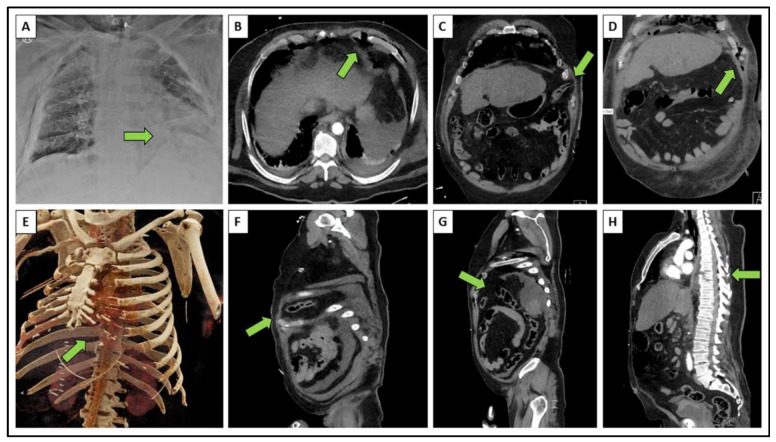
Initial X-ray and whole body CT scan: (A) anterior-posterior view, (B) axial CT slice, (C,D) coronar CT slices, (E) 3D-CT reconstruction, (F–H) sagittal CT slices. The respective injuries are marked with arrows. A 65-year-old male suffered from a fall from a height of six meters. Initial X-ray of the chest shows left-sided high-riding diaphragm (Figure 1A). CT scan proves anterior cartilage fracture (Figure 1B), traumatic intercostal hernia within the widened 7th intercostal space (Figure 1C–F), disruption of the diaphragm creating a two-cavity injury (Figure 1G), and distraction fracture of the 7th thoracic vertebra (Figure 1H). The diagnostics showed additional ipsilateral serial rib fractures posterolateral with involvement of the ribs VI–X. This results in a combined thoracovertebral injury entity, consisting of a disruption of the thoracic spine and the costal arch monolaterally torn in the anterior area with anterior fractures of the ribs VII and VIII at their cartilage conjunction, in addition to their posterolateral fractures. The sternum, the mediastinal organs, the center of the diaphragm, and the great vessels did not show any injuries in this case.
